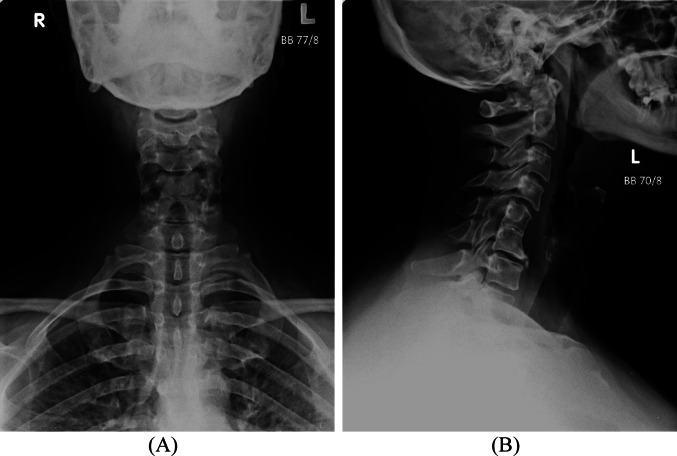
A-B Anteroposterior (A) and lateral (B) radiographic views of the cervical spine demonstrating Klippel-Feil syndrome at the C5–C6 level, characterized by congenital fusion of the vertebral bodies with loss of normal intervertebral disc space and reduced cervical mobility
